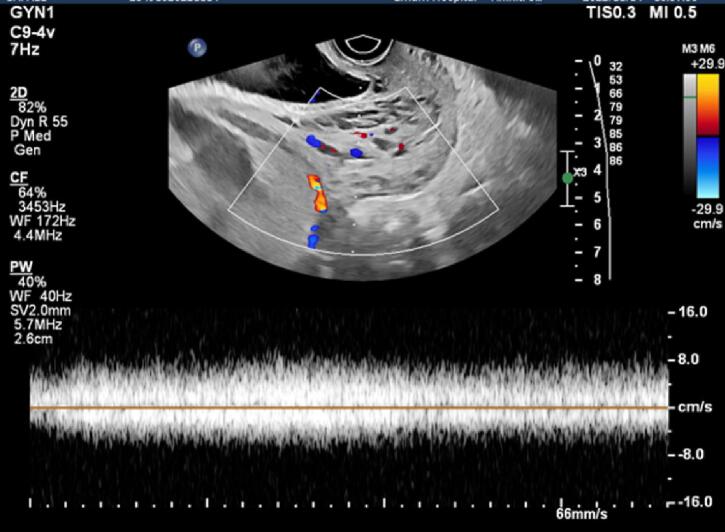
Color Doppler sonography showed venous flow in cervical varices.
